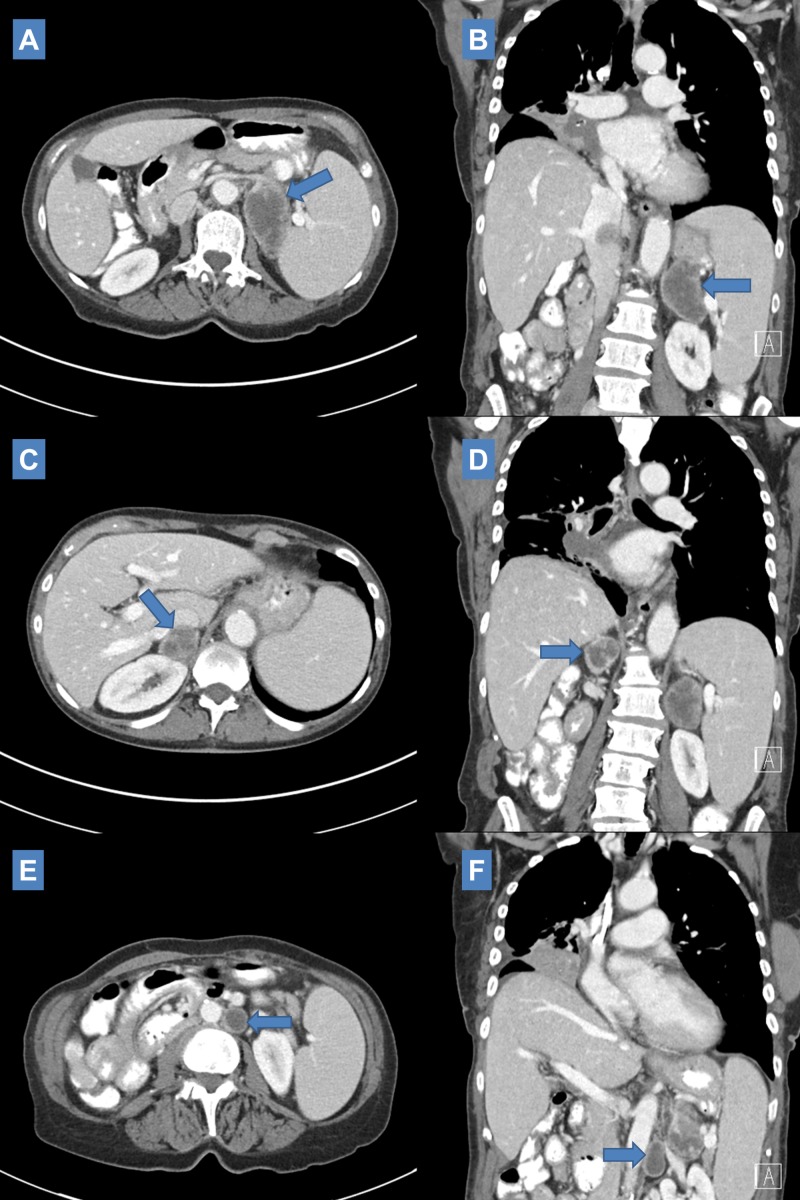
Three-month post definitive treatment computed tomography (CT) scan visualizing enlarging left adrenal metastasis (6.4 cm x 3.7 cm
